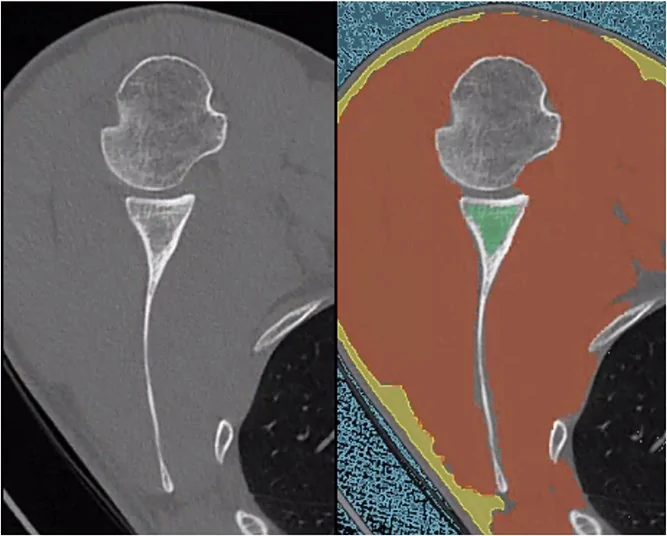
Axial CT slice of a right shoulder. Original gray-scale image on the left, with the corresponding referene tissue segmentations on the right, demonstrating air (blue), fat (yellow), muscle (red) used for density calibration of the glenoid trabecular bone (green). The air region of interest (RoI) was semi-automatically segmented based on defined thresholds (–1023 HU to –970 HU; –1024 (lowest detectable HU number) was spared to prevent inclusion of empty image regions). The muscle ROI was automatically segmented using TotalSegmentator, while the subcutaneous fat and glenoid ROIs were automatically segmented using internally trained deep-learning models. This single axial slice is shown for visual purposes; all reference tissues were segmented in the entire CT scan, where anatomically present.
